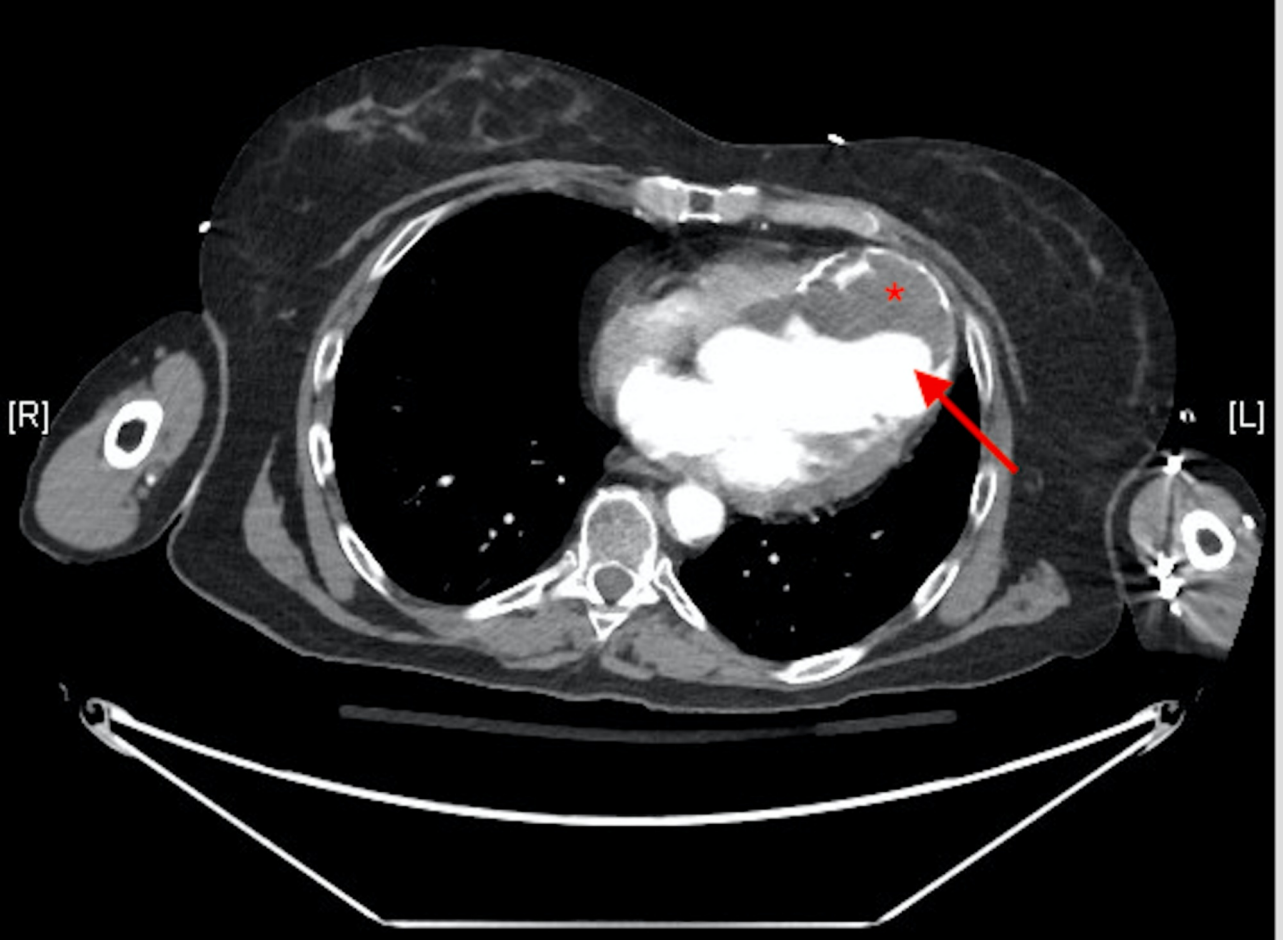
Contrast-enhanced CT, axial section - a large mass is seen adhering to the anterior left ventricular wall suggestive of a large mural thrombus (asterisk) within the left ventricular aneurysm (arrow).
